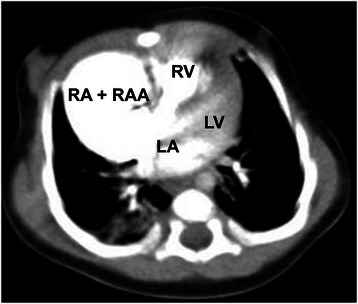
Heart CT scan. A CT of the patient’s chest at the time of diagnosis. The patient was 2 month old. The size of the aneurysm was 3.9x3.4x3.4 cm. RA + RAA indicates aneurysmatic right atrium. RV indicates right ventricle, LA – left atrium, LV – left ventricle
